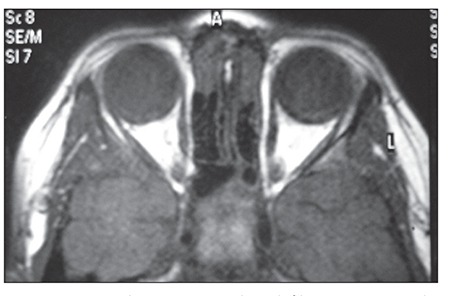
MRI shows crescent-shaped fibrotic tissue in theposterior region of the left bulbus oculi, which was considered calcification without evidence of a mass.
